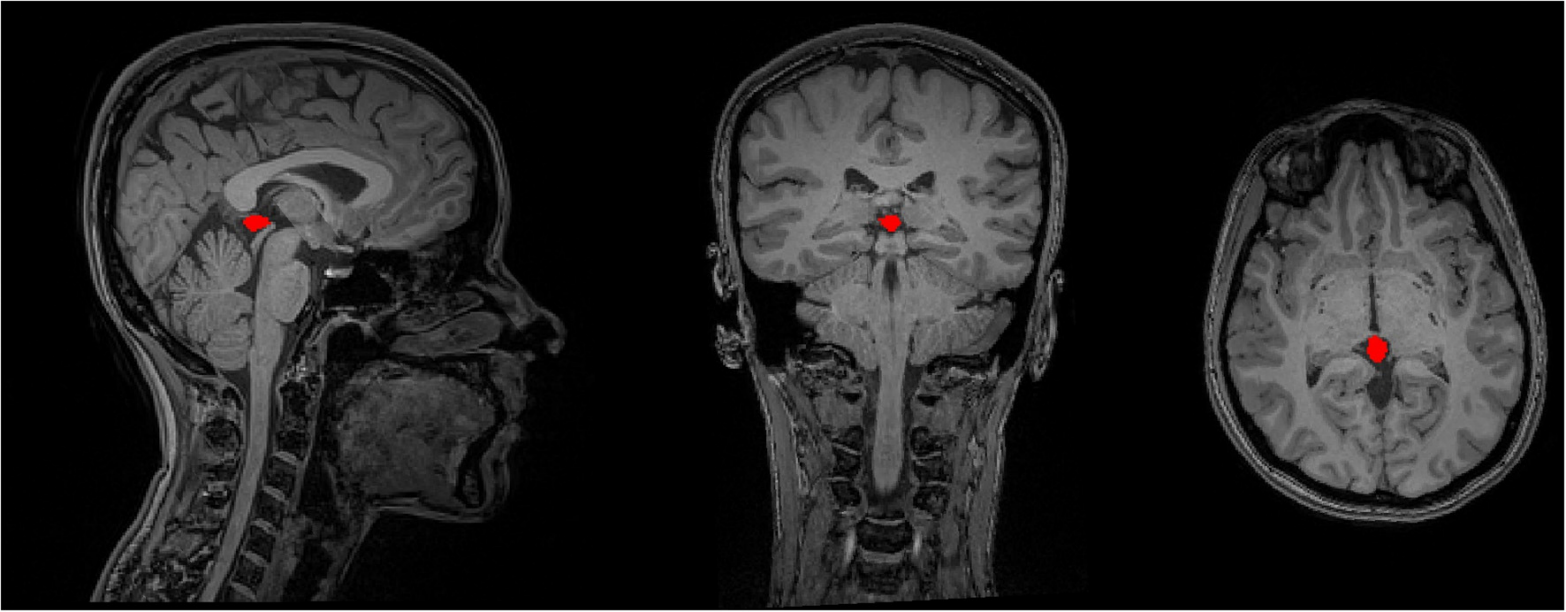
The delineation of the PG's contour on a sample T1-weighted MRI data in the sagittal, coronal, and axial image views.
